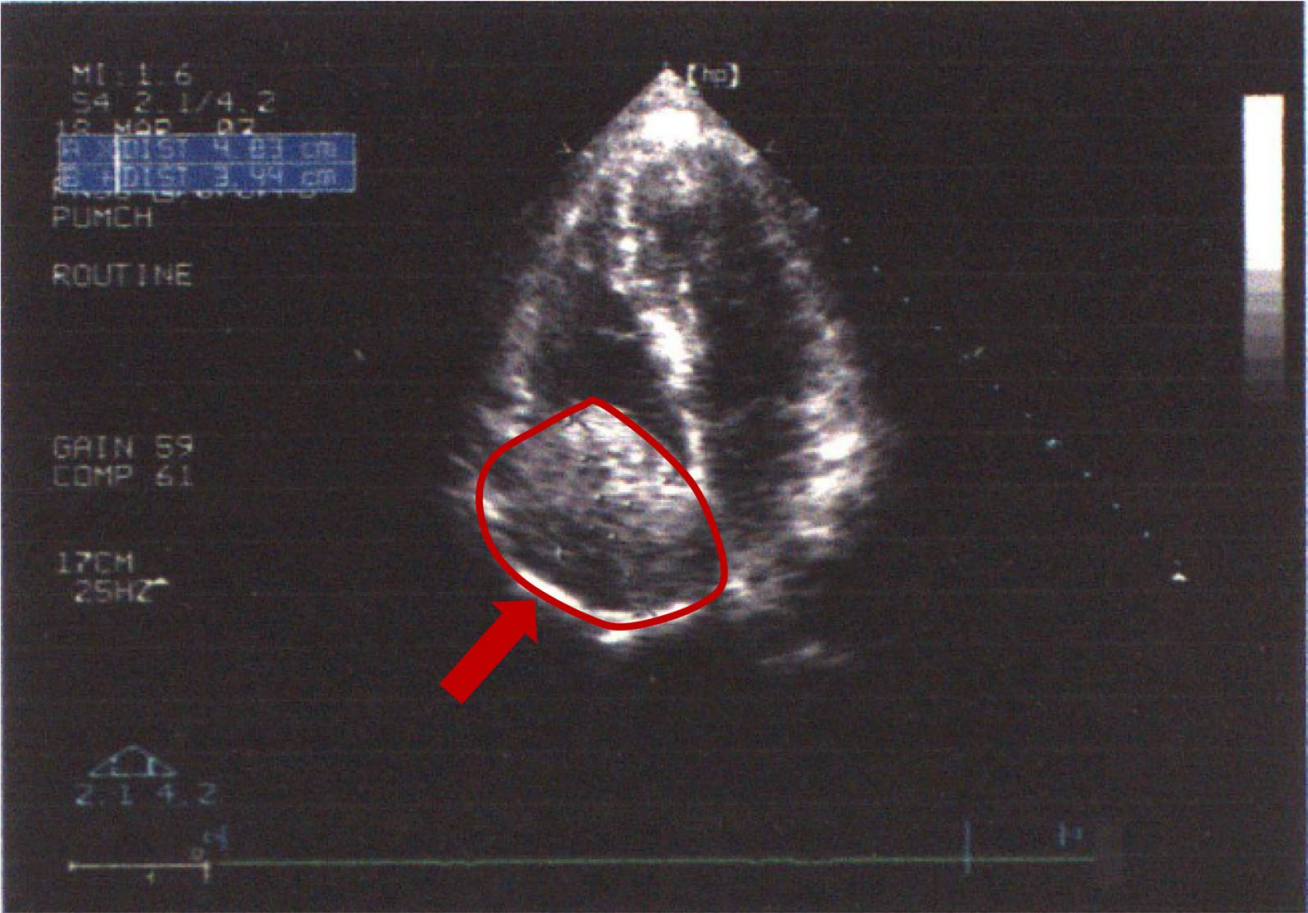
Echocardiogram showing an intracardiac tumor located in the right atrium (red arrow)
